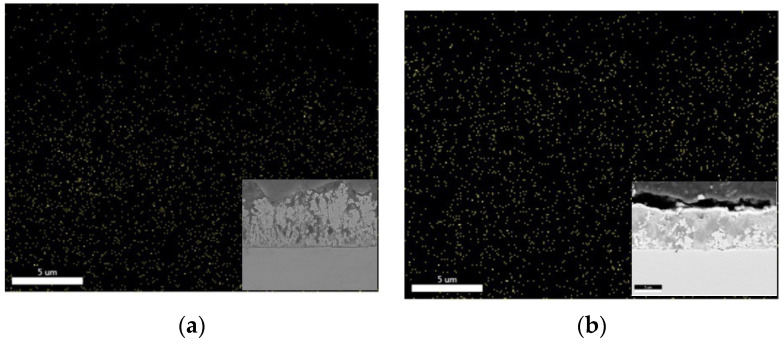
EDAX mapping of cerium in Zn-Co-CeO_2_ coatings deposited under ultrasound at 2 A dm^−2^ from solutions containing (a) 0.2 wt.% CeO_2_ powder and (b) 0.2 wt.% CeO_2_ sol, all elements. Cross-section SEM images are shown as inserts.
